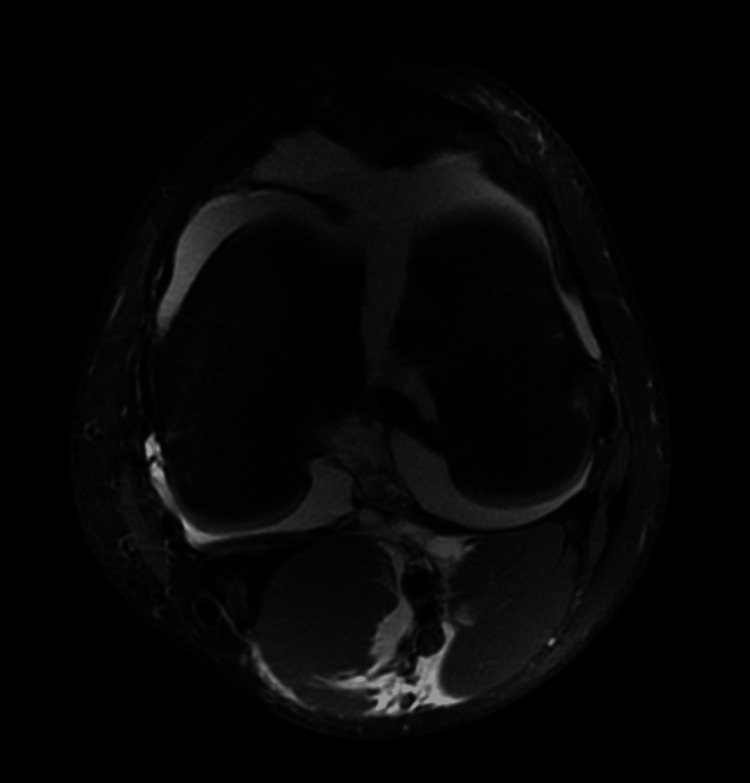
MRI of the left knee, transverse view
